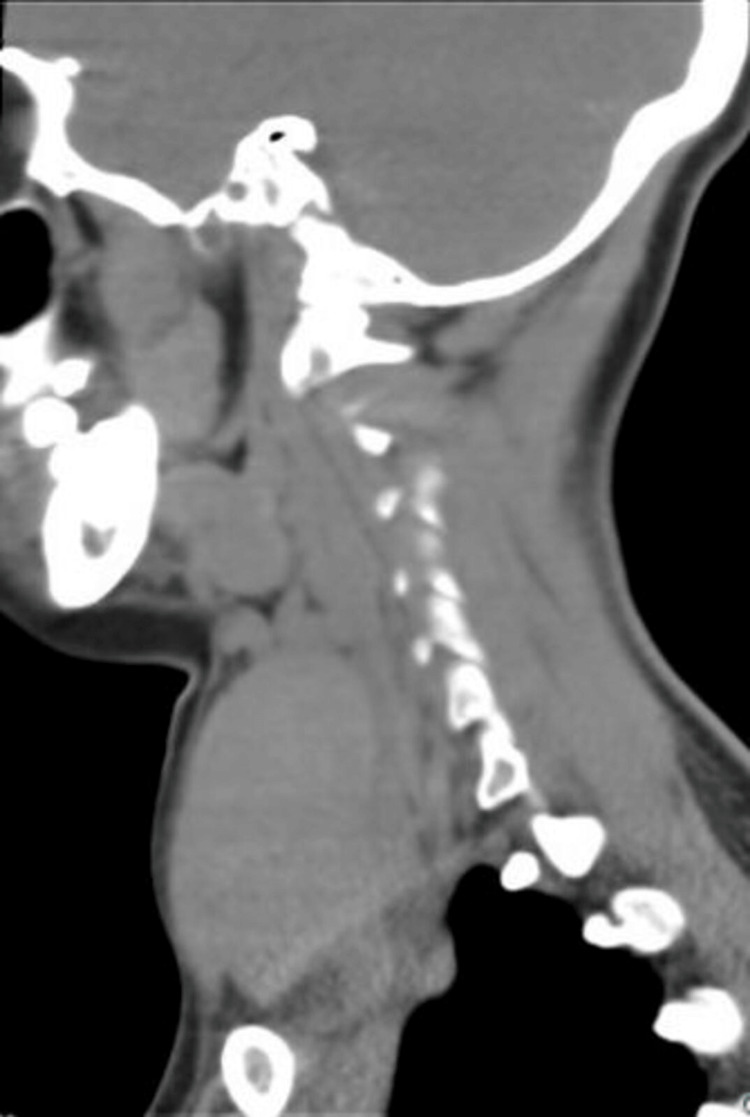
Sagittal CT reconstruction demonstrating diffuse enlargement of the thyroid gland.
